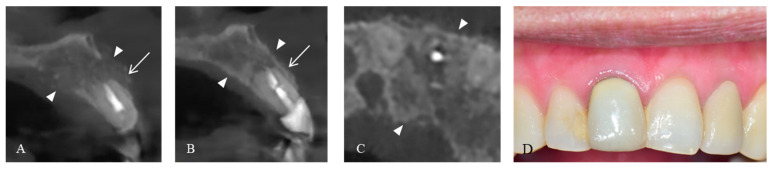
Postoperative images. (A) After one week, M-DDM was observed to have been successfully grafted onto the cystic defect in the area of cortical bone loss (arrowhead) and on the alveolar bone above the maxillary left lateral incisor (arrow). (B) M-DDM had healed into the cortico-cancellous complex, resulting in intact bucco-palatal cortical bone (arrowhead) and a significant increase in the thickness of the buccal alveolar bone around the maxillary left lateral incisor (arrow). (C) The axial view of CBCT also showed bone healing with cortical lining on both bucco-palatal contour (arrow). (D) The soft tissue around the maxillary left lateral incisor showed intact healing with sufficient volume.
